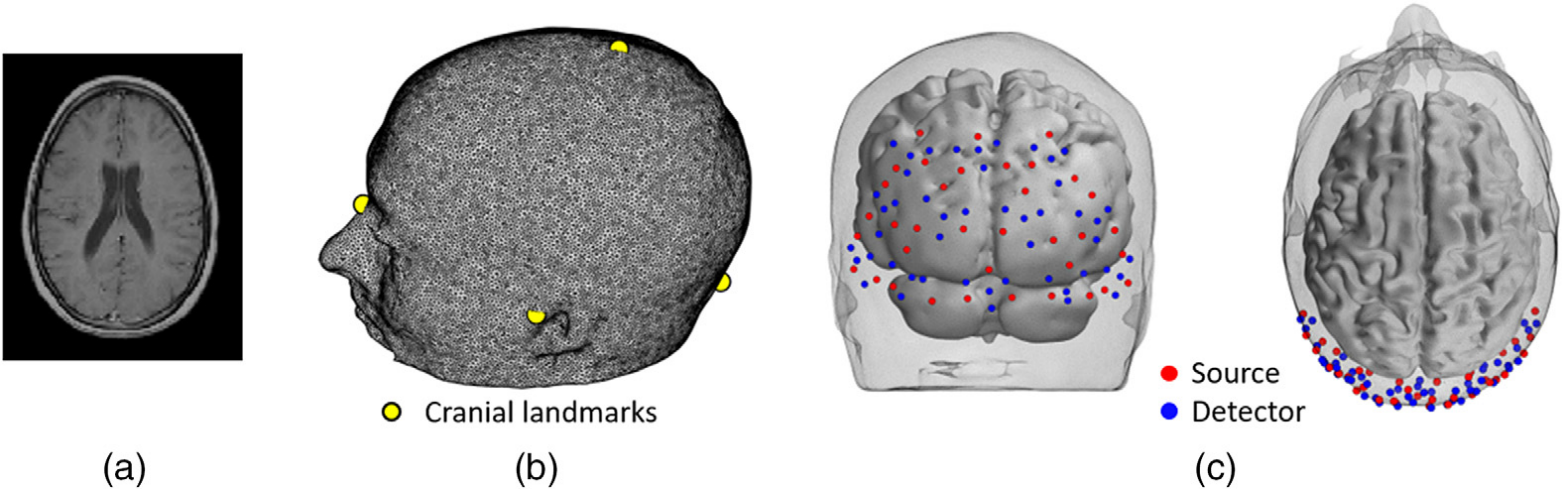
(a) Anatomical MRI; (b) high-resolution tetrahedral mesh, landmarks are indicated with the yellow circles; and (c) 3D model used for image reconstruction. Red and blue circles denote source and detector locations.
